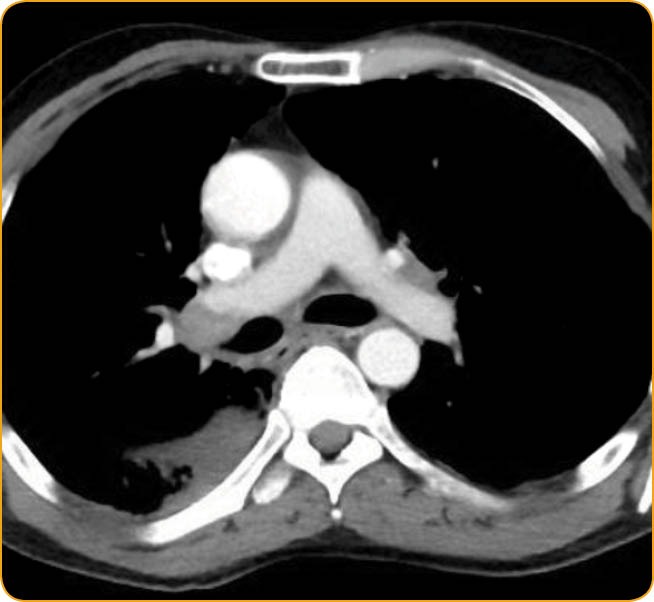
CT chest/abdomen window shows the enlarged right hilar lymph node.
